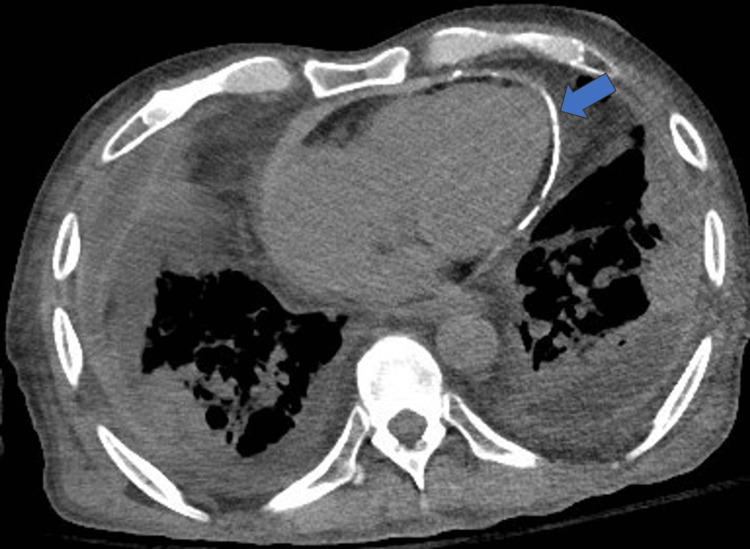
CT chest without IV contrast showing pericardial calcifications (blue arrow)
